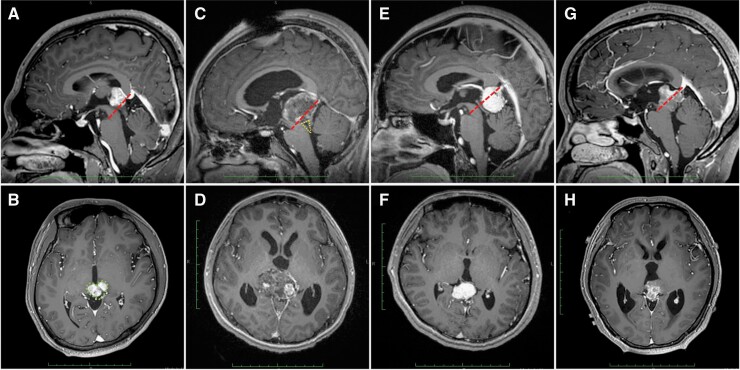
Representative sagittal (upper row) and axial (lower row) T1-weighted contrast-enhanced magnetic resonance images obtained from 4 patients. (A and B) A 21-year-old male patient with germinoma. (C and D) A 14-year-old male patient with mature teratoma. (E and F) A 39-year-old female patient with pineal parenchymal tumor of intermediate differentiation. (G and H) A 29-year-old female patient with pineoblastoma. The red dotted line indicates the G-I line (the line between origin of the Galen vein and lowest point of the interpeduncular cistern). The green dotted line delineates the cardioid shape sign. The yellow dotted line delineates the trumpet sign.
